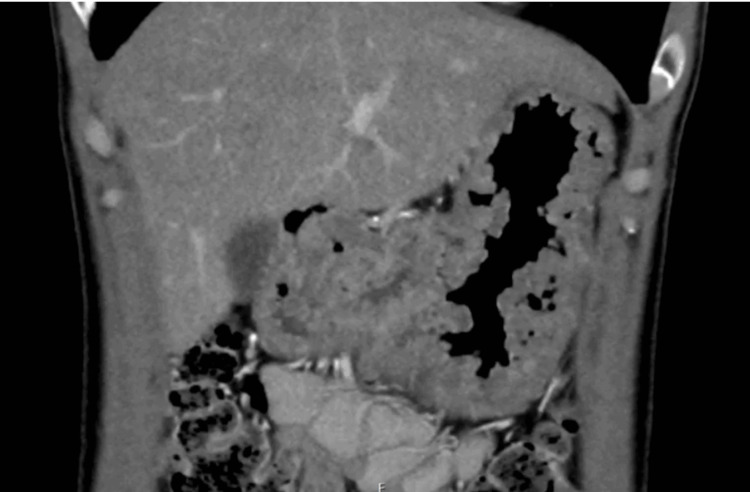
The image of computed tomography (CT) finding CT reveals wall thickening of the stomach and numerous polyp-like protrusions.
